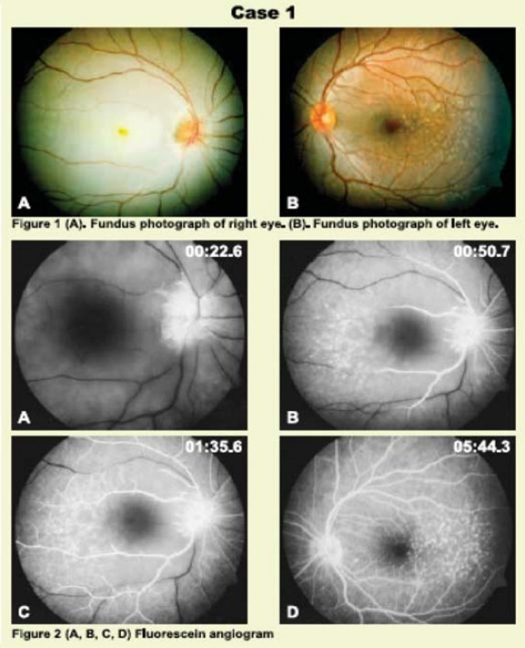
Fundus examination and fluorescein angiography of Case 1.Cardiac evaluation was performed by a cardiologist and her carotid doppler was within normal and echocardiography showed mild mitral regurge. The patient was treated with Aspirin.
